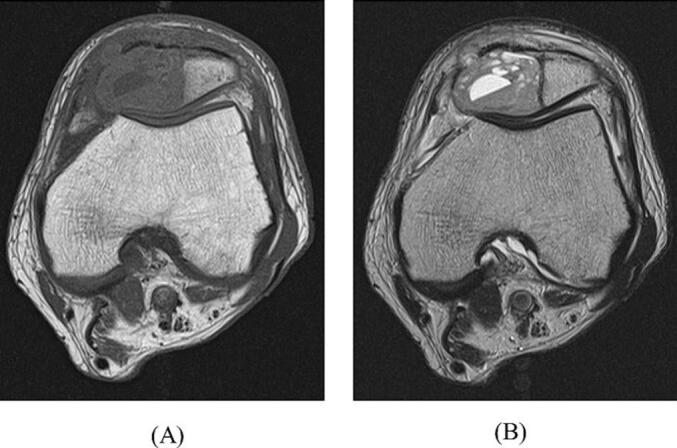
Preoperative images of a patellar tumor of the left knee. Magnetic resonance image of the left knee in the axial view. (A) T1-weighted image showing a lesion occupying the medial side of the patellar bone marrow. (B) T2-weighted image showing multifocal fluid formation within the patellar bone marrow, with some tumors infiltrating outside the patella.
